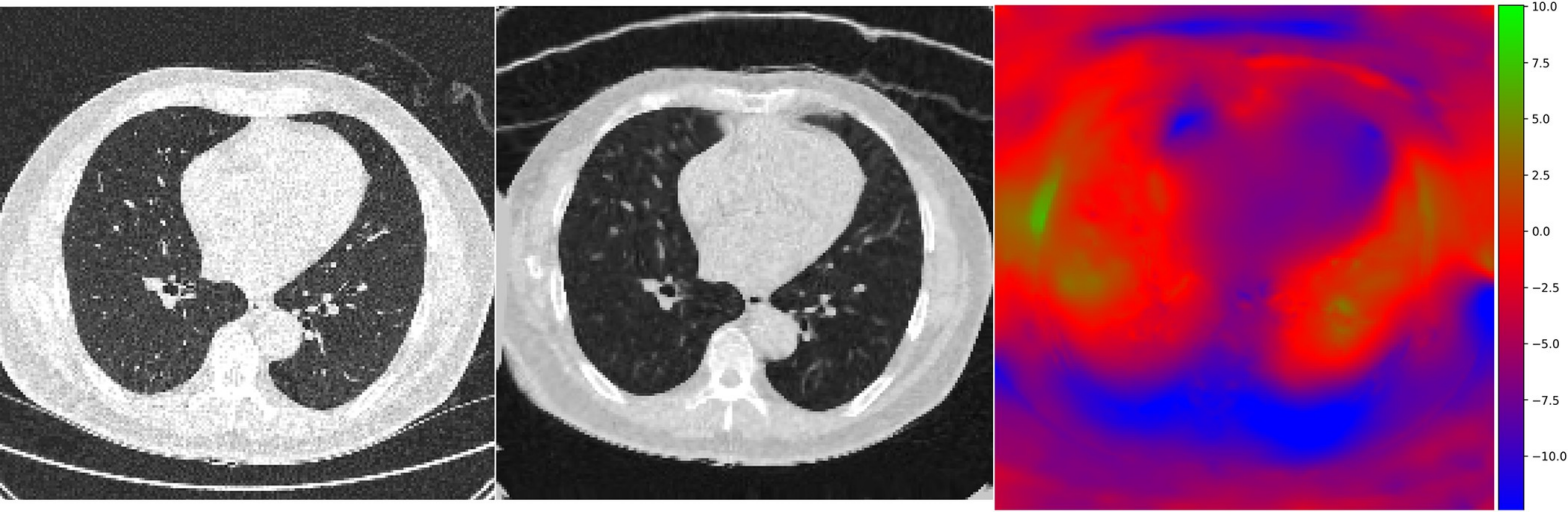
Representative pixel movement in deformable registration between supine and prone CT scans in a 71-year-old man without pulmonary fibrosis. Using ANTsPy, the CT images were resized into 192×192×192 resolution. After affine registration and deformable registration of both supine (A) and prone (B) images, the spatial displacement map was calculated. Whole-lung movement between the supine and prone positions was measured by the 3D Euclidean distance in millimeters. On a HSV map (C), there are variable movements in bilateral lungs, as color coded ranging from green to red. Note.–ANTsPy: Advanced Normalization Tools in Python package, 3D: three-dimensional, HSV: hue-saturation-value.
